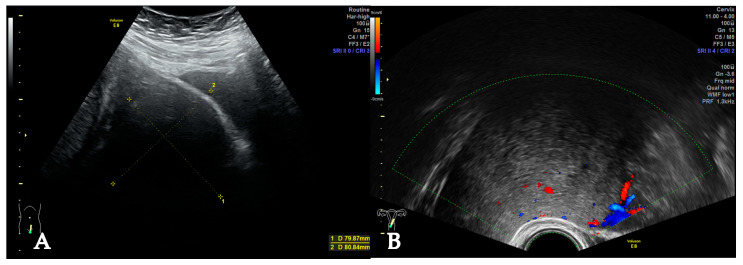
Uterine mass during pregnancy. Abdominal ultrasound at 38 weeks (A). Ultrasonographic findings were suggestive of deviation of the labour canal, especially as seen in PU (B). b—bladder, m—mass.
